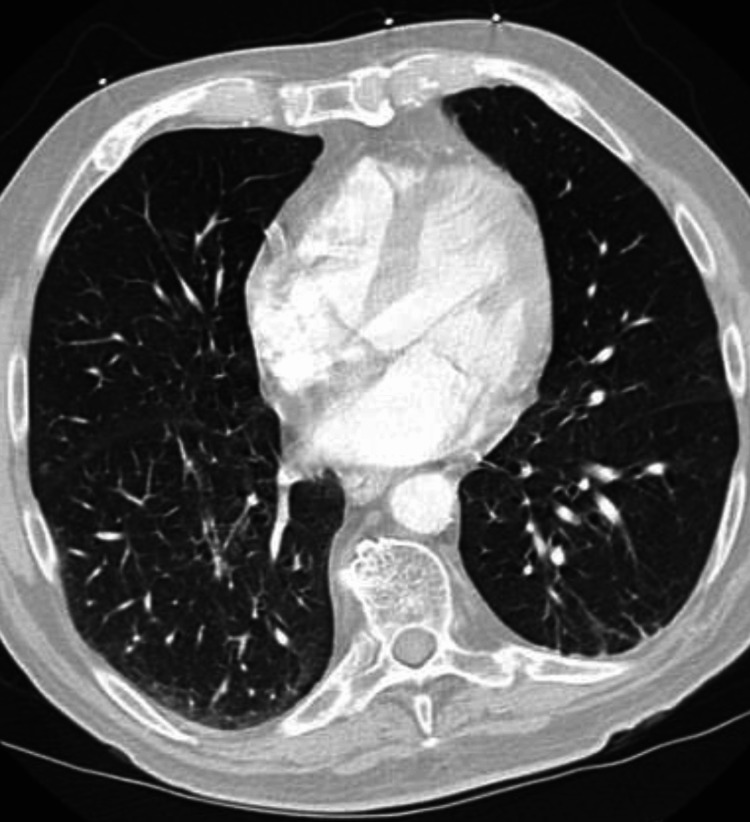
CT Angiogram of Chest Official read of lungs: Faint heterogeneous opacity of the right lung which is favored chronic.
